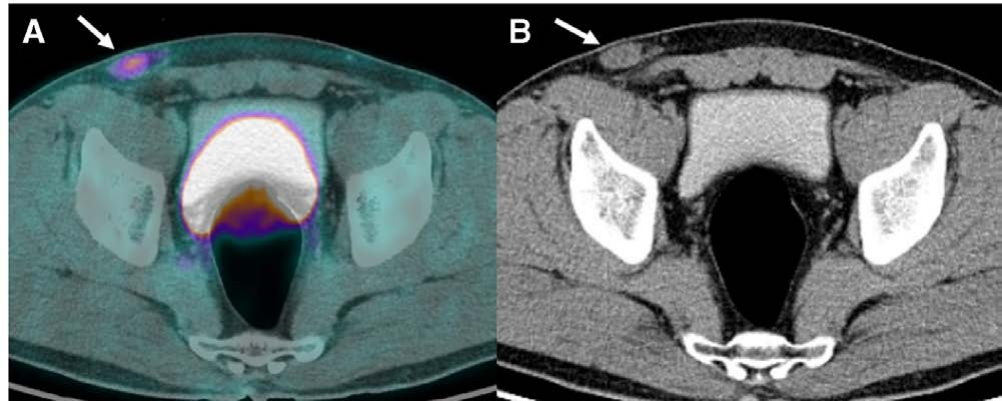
(A and B) FDG PET/CT demonstrates an oval nodular structure in the right inguinal area (arrows) with moderate FDG uptake (SUV_max_ = 4.10). FDG = fluorodeoxyglucose, PET/CT = positron emission tomography–computed tomography, SUV = standardized uptake value.
